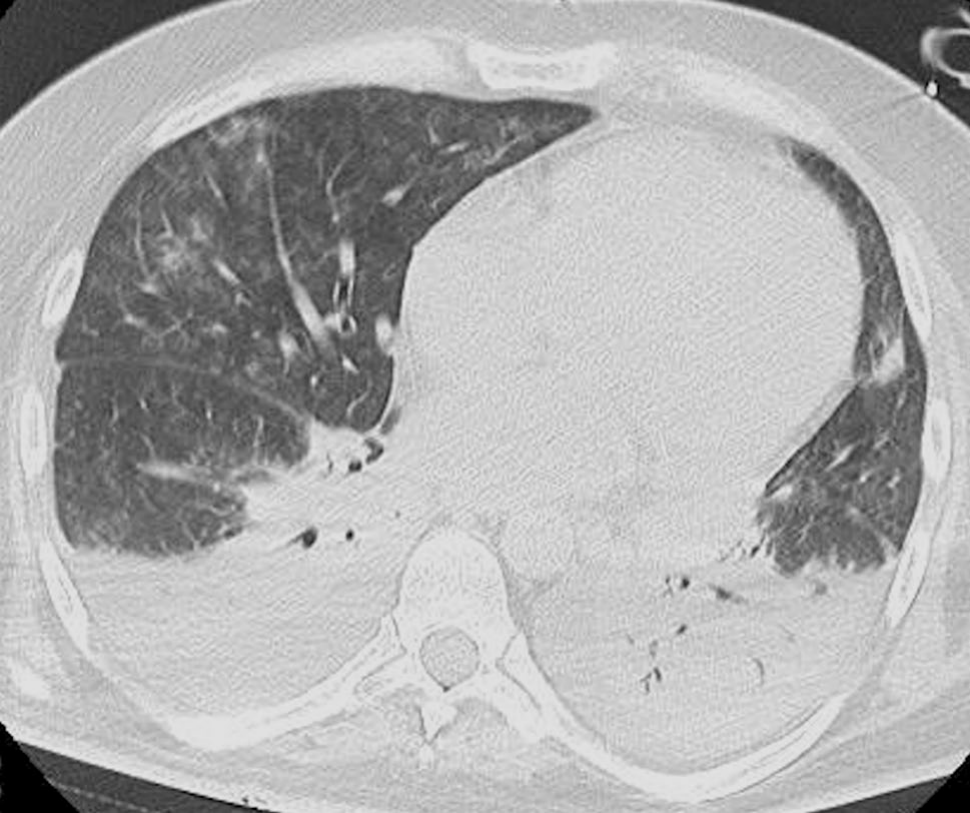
Axial CT image demonstrating bilateral lung consolidation in a COVID-19 patient with New Delhi Metallo-β-Lactamase (NDM)-producing Klebsiella pneumoniae
